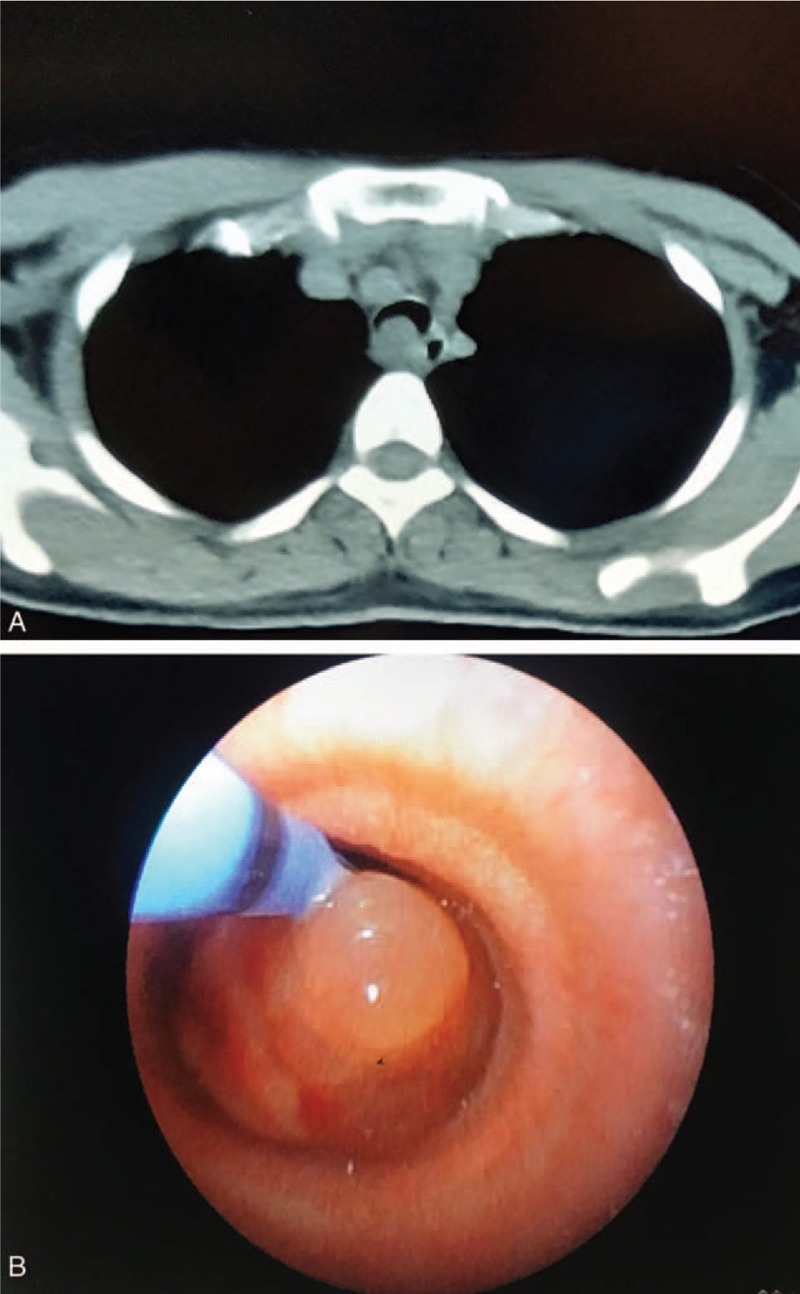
Intratracheal schwannoma on CT scan and bronchofibroscopy examination before first endoscopic surgery. A: Chest CT showing a ∼1.5 cm intratracheal tumor (arrow), located on the right lateral posterior wall of the trachea at the level of the brachiocephalic veins. B: Bronchofibroscopy revealing a whitish, round mass with a wide base, nearly obstructing about 90% of the tracheal lumen.
